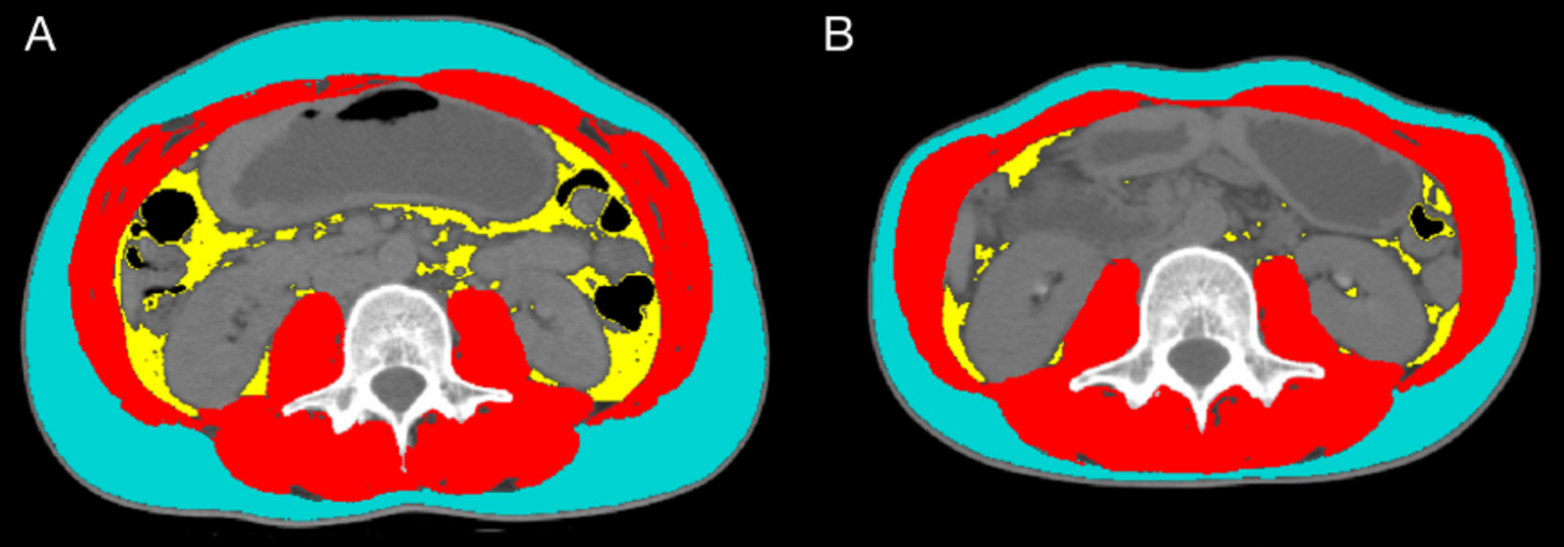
Cross‐section of pre‐NAC and post‐NAC CT‐scan images at the L3 region from the same patient. (A) Pre‐NAC. (B) Post‐NAC. Red: skeletal muscle; blue: subcutaneous adipose tissue; yellow: visceral adipose tissue. CT, computed tomography; NAC, neoadjuvant chemotherapy.
